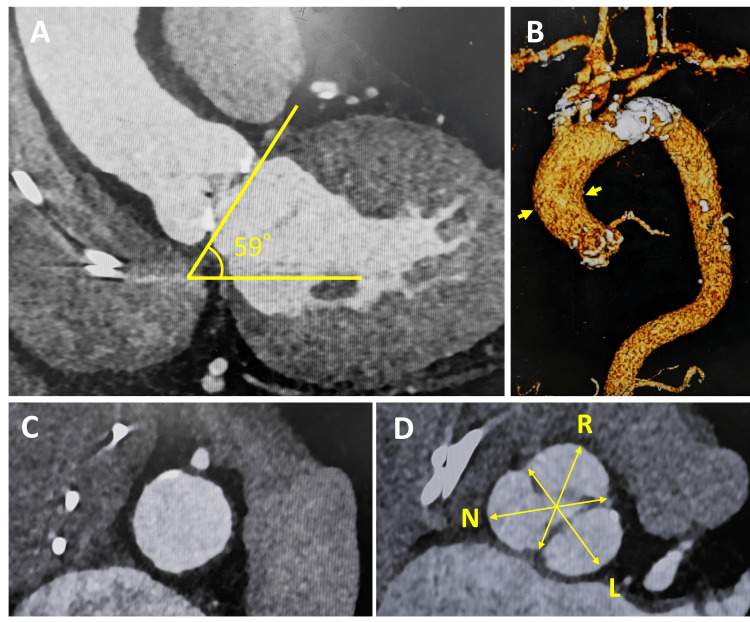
Findings on preoperative computed tomography (A) The aortic root demonstrated an angulation of 59°. (B) A sharp curvature extending into the proximal ascending aorta (yellow arrow) . (C) Minimal calcification at the sinotubular junction. (D) The aortic valve was tricuspid, and the sinus of Valsalva diameters were 25.6 mm (R: right coronary cusp), 27.9 mm (L: left coronary cusp), and 27.9 mm (N: non-coronary cusp).
